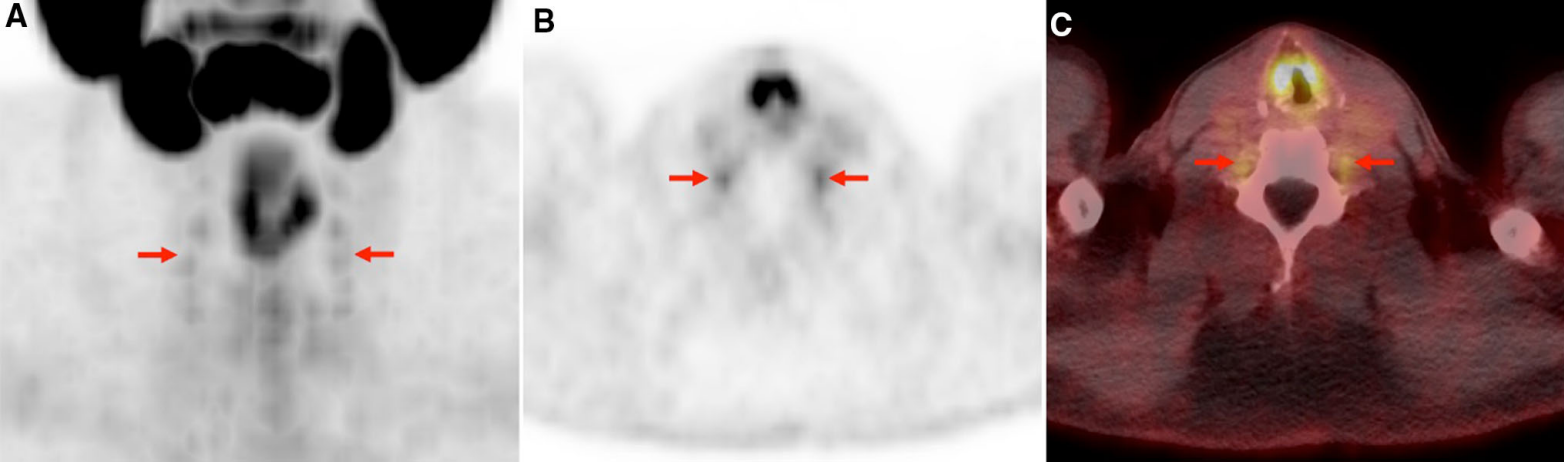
a Anterior view of ^18^F-DCFPyL maximum intensity projection (MIP) image from a 56-year-old PCa patient undergoing preoperative staging. Mild radiotracer uptake is seen in multiple cervical DRG bilaterally (*red arrows*). b Axial ^18^F-DCFPyL PET and c axial ^18^F-DCFPyL PET/CT fusion images from the C5–6 level also show mild radiotracer uptake in the C6 DRG bilaterally (*red arrows*). Note normal physiologic biodistribution of this radiotracer including uptake in the major salivary glands and structures of the larynx
